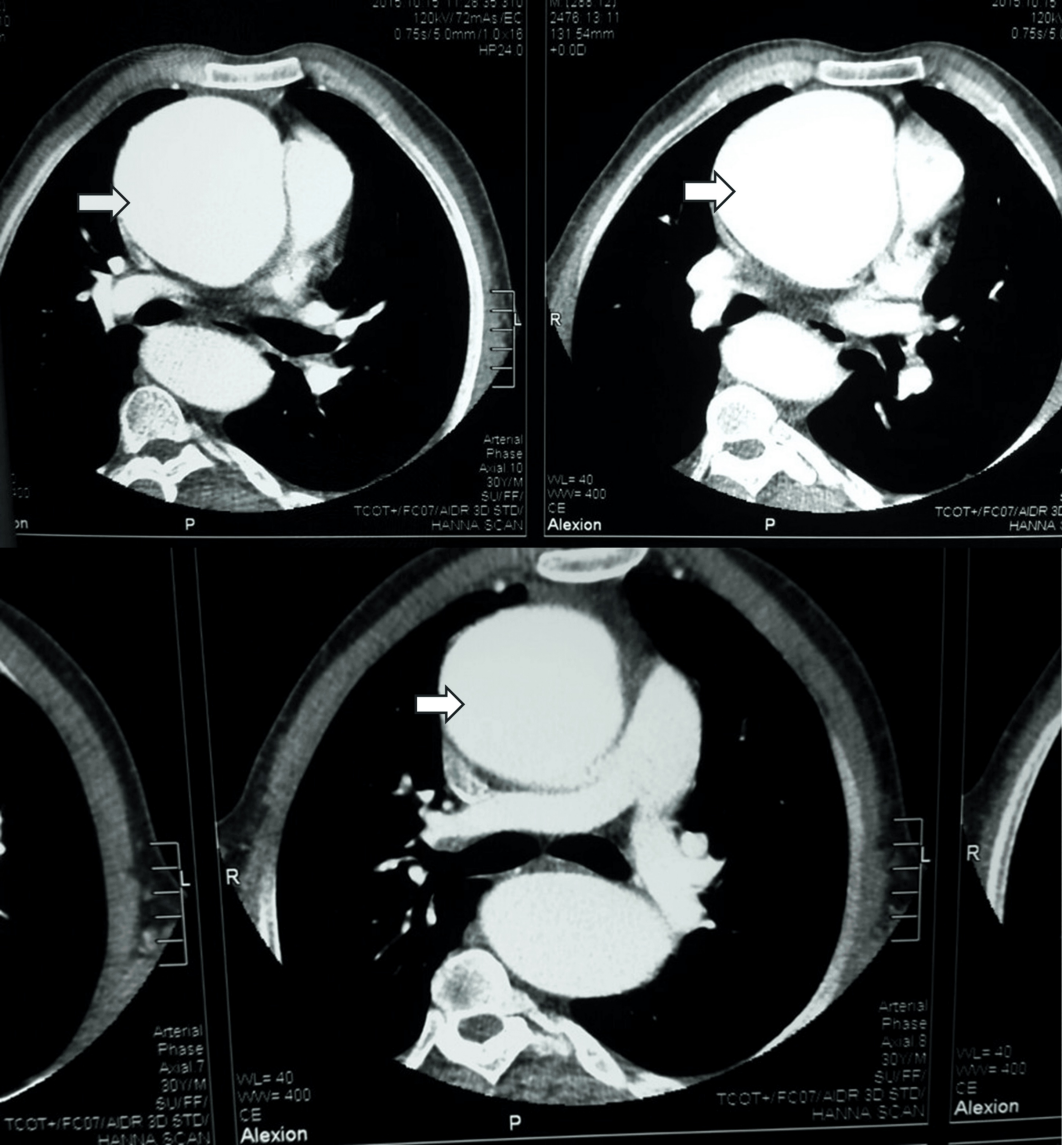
Arrows indicate large ascending aortic aneurysm in CT aortography
